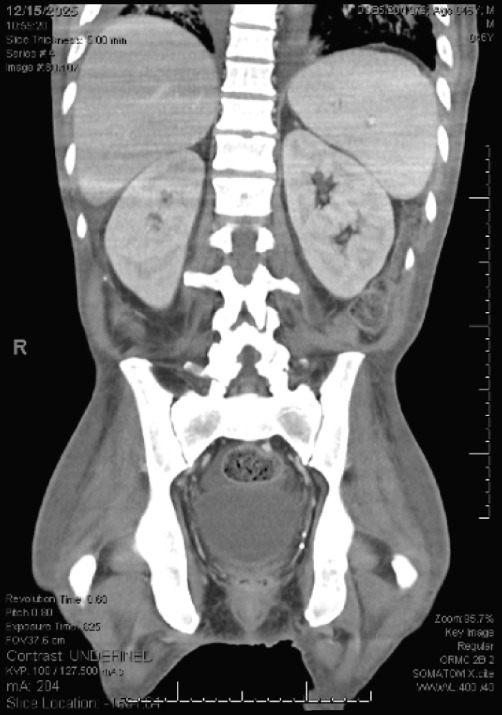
CT abdomen and pelvis (coronal view) demonstrating splenomegaly (138 mm).
